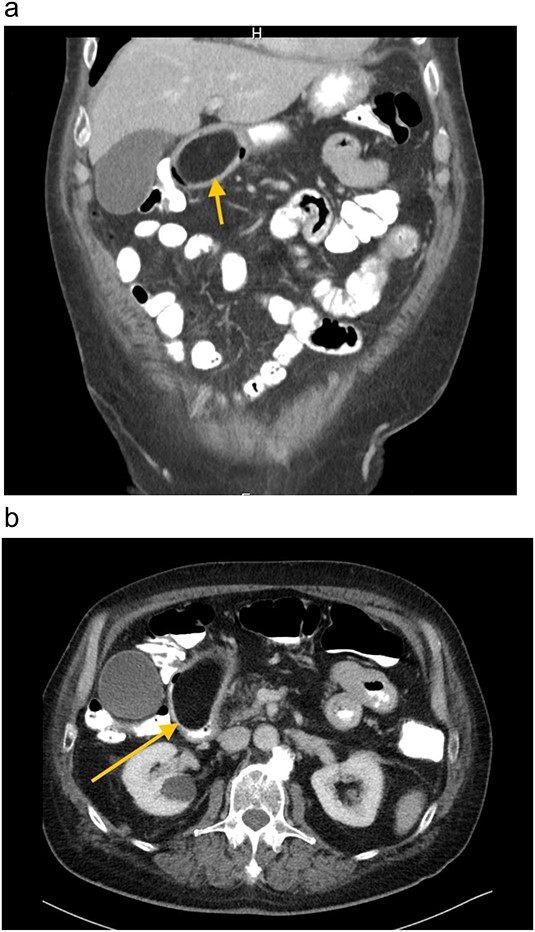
(a) and (b) CT-Abdomen, showing duodenal mass ⁓7.4 × 4.4 cm pressing the Pylorus.
